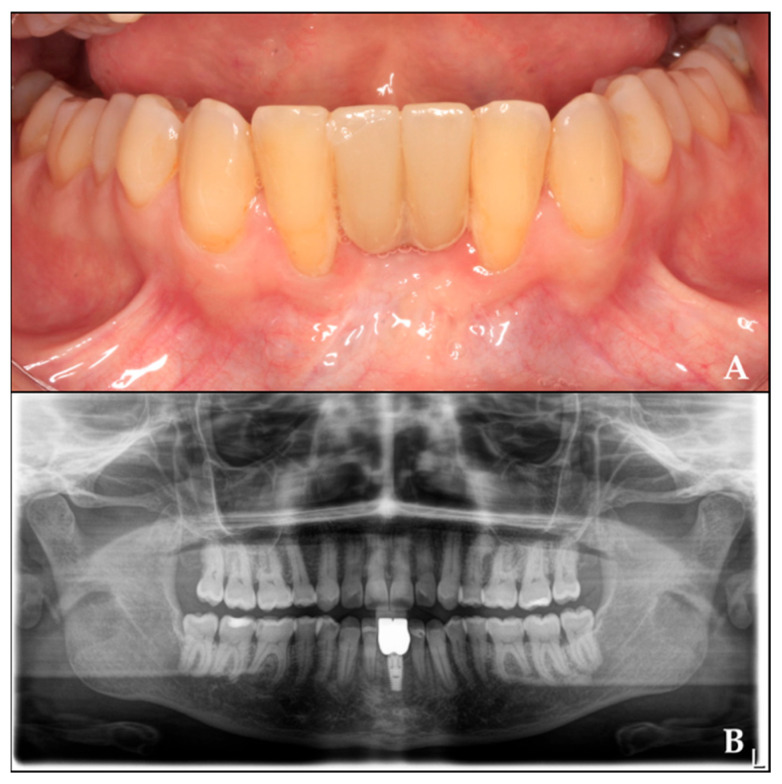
Rehabilitation of the regenerated area with crowns twenty months after implant placement. (A) Prosthetic rehabilitation in the regenerated area. (B) Orthopantomography with crowns over the implant.
